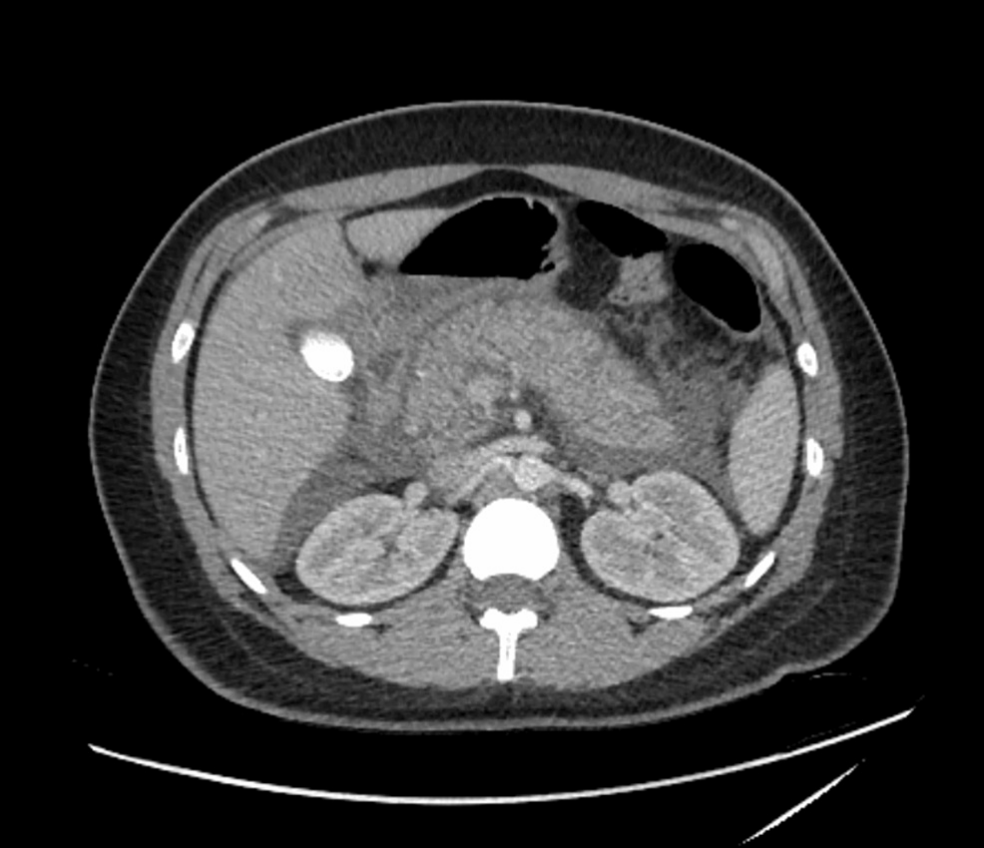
CT showing hemorrhagic pancreatitis
